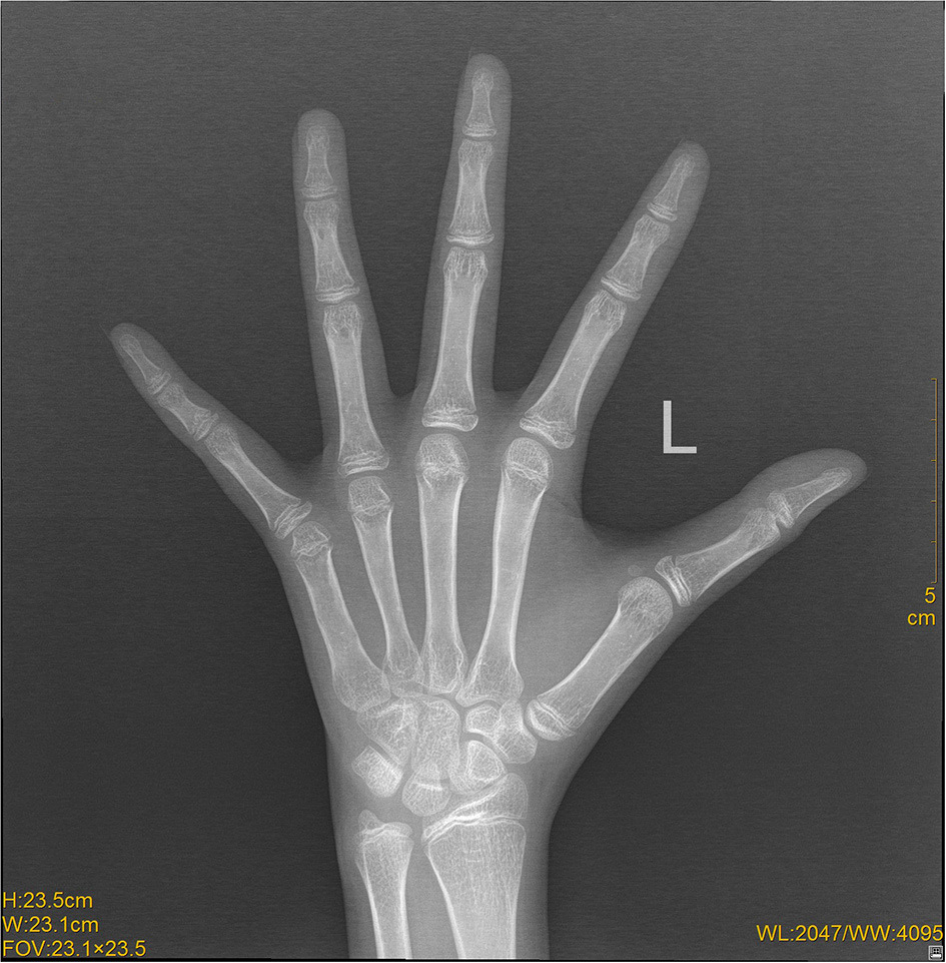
X-ray image of the left wrist. X-ray showed that the bone age was 13 years old.
